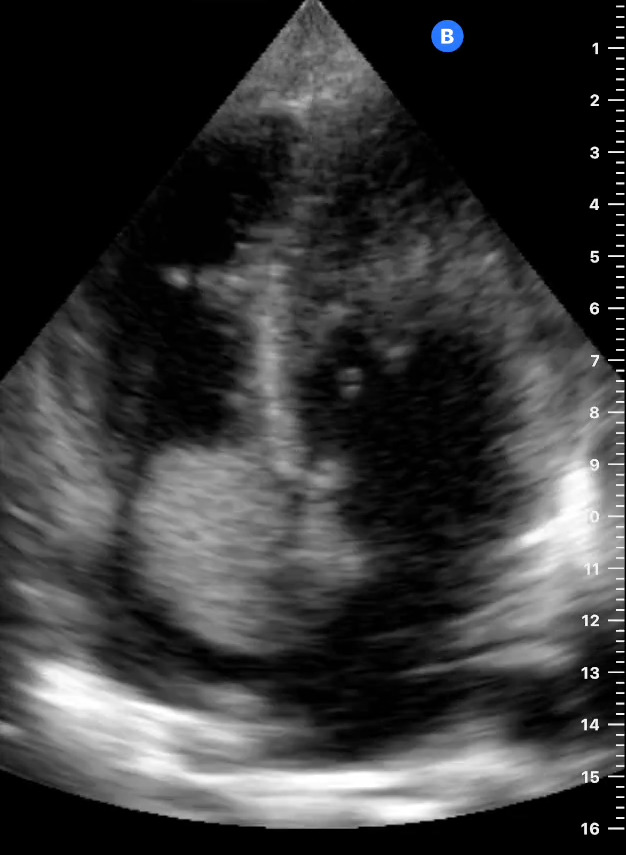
Apical four-chamber view during diastole showing a 4x2 cm right atrial mass (arrow) originating from the interatrial septum with almost complete obstruction of tricuspid valve during atrial systole.
